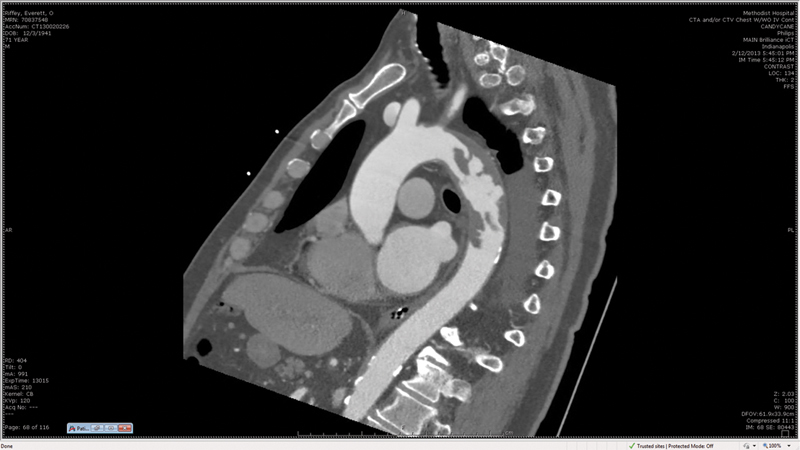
Computed tomography scan image.
